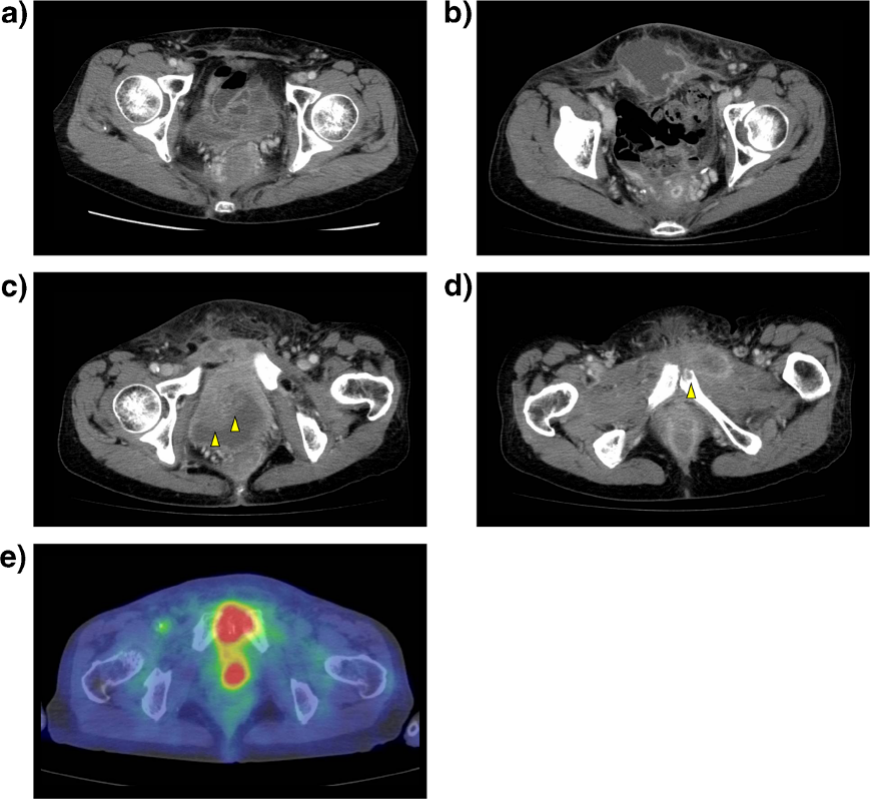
Postoperative imaging modalities. a) Computed tomography (CT) 6 months after surgery. No abscess is seen. b-d) CT 1 year after surgery. A marginally enhanced low-density mass lesion is seen in the lower abdominal wall (b). The mass lesion is adjacent to the bladder wall with bladder wall thickness (c, yellow arrow) and the pubic bone with partial bone destruction (d, yellow arrow). The tumor shows high uptake on positron-emission tomography (e).
